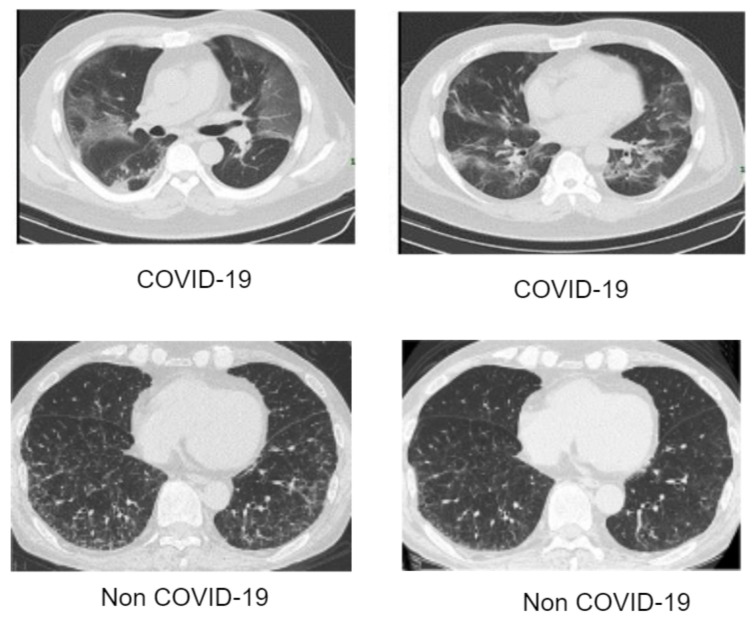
Data collection of computed tomography (CT) images of COVID-19 and non-COVID-19.
